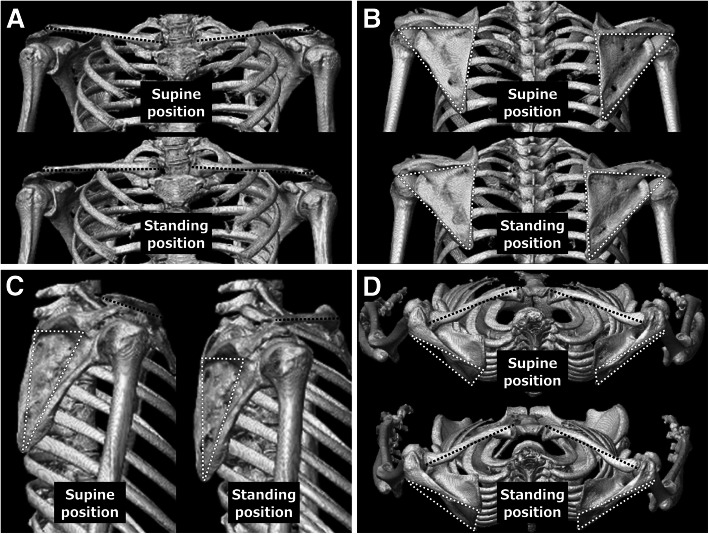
Three-dimensional CT scans in the supine and standing positions. a Anterior view. Compared with the supine position, elevation of the clavicle (black dotted lines) decreases in the standing position due to the effect of gravity. b Posterior view. Compared with the supine position, upward rotation of the scapula (surrounded by white dotted lines) decreases in the standing position due to the effect of gravity. c Lateral view. Compared with the supine position, the scapula (surrounded by white dotted lines) is located inferiorly and tilts posteriorly in the standing position. d Superior view. Compared with the supine position, the clavicle (black dotted lines) shows greater retraction, and the scapula (surrounded by white dotted lines) is located medially and rotates externally in the standing position
